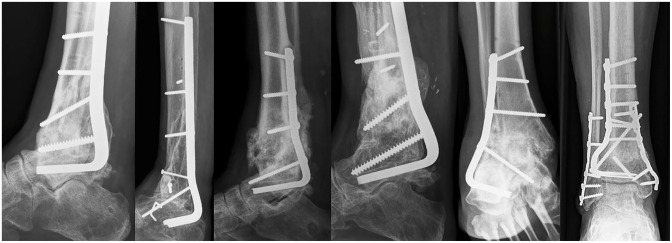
Final follow-up radiographs of 6 representative cases (4 posterior and 2
